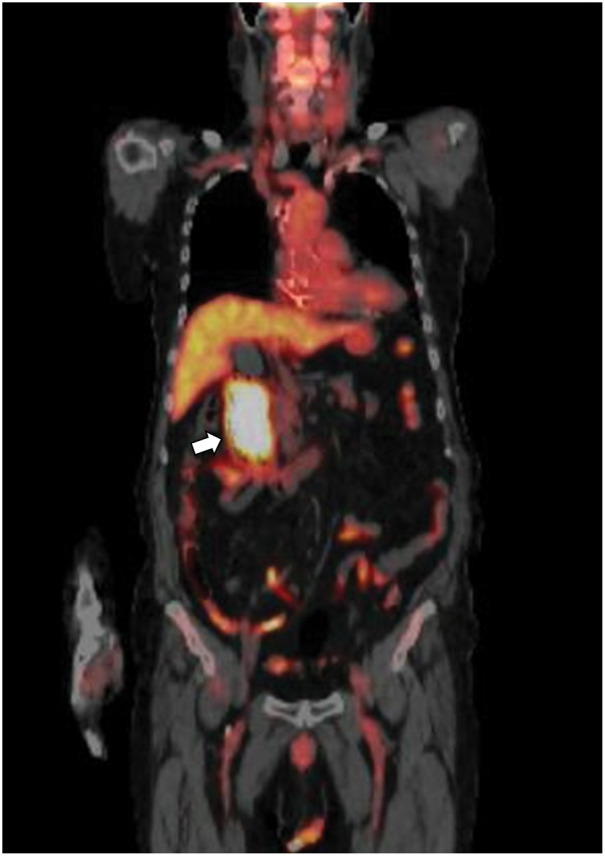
PET-CT. The mass lesion demonstrated abnormal FDG uptake with a maximum standardized uptake value of 6.57. FDG, fluorodeoxyglucose
